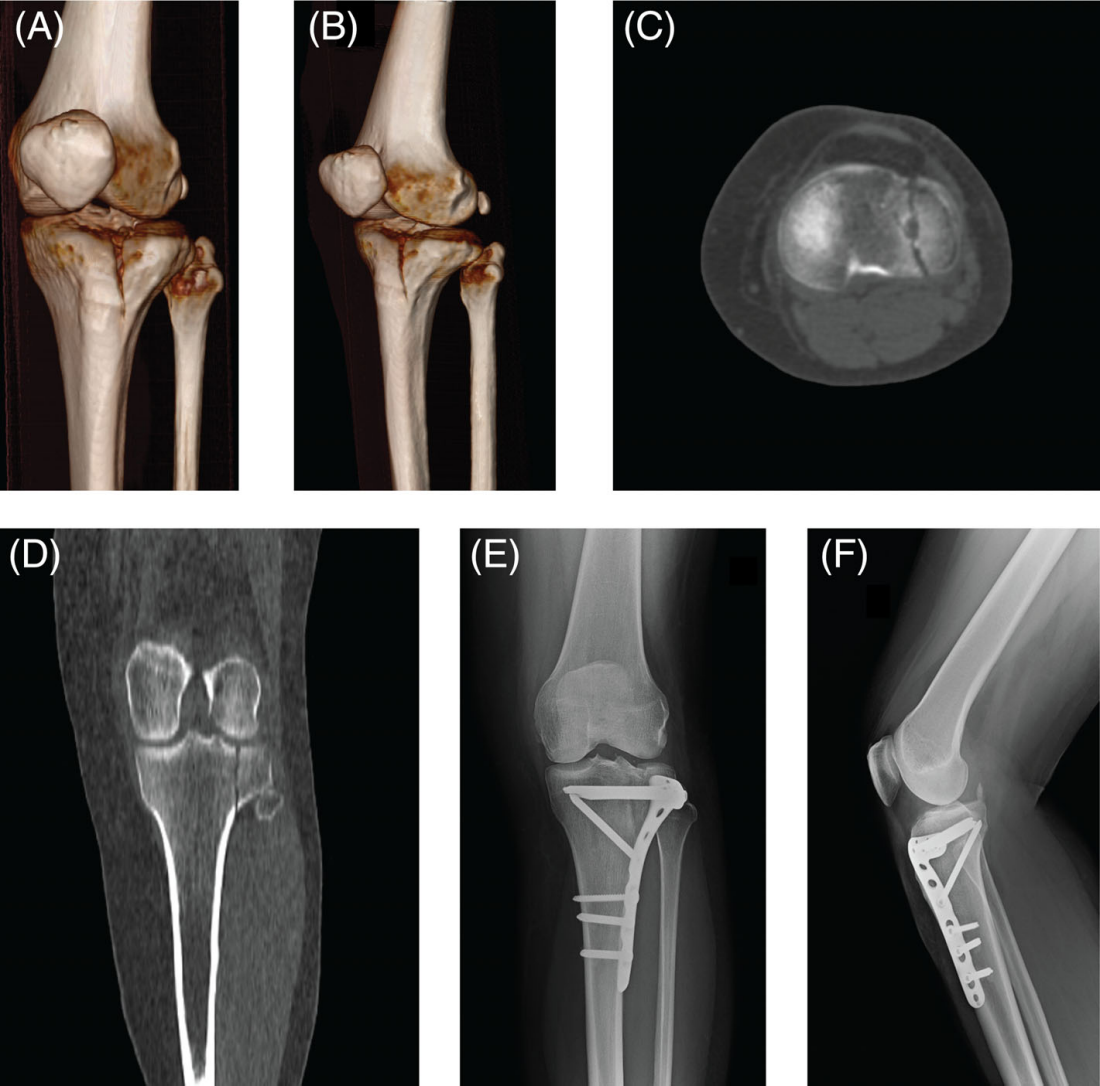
Case 2: A 75‐year‐old woman with an AO 41‐B1 fracture. (A, B) Preoperative 3D‐CT scan reconstruction images; (C) CT axial image; (D) CT coronal view; (E, F) Anteroposterior and lateral radiographic images 6 months after surgery.
